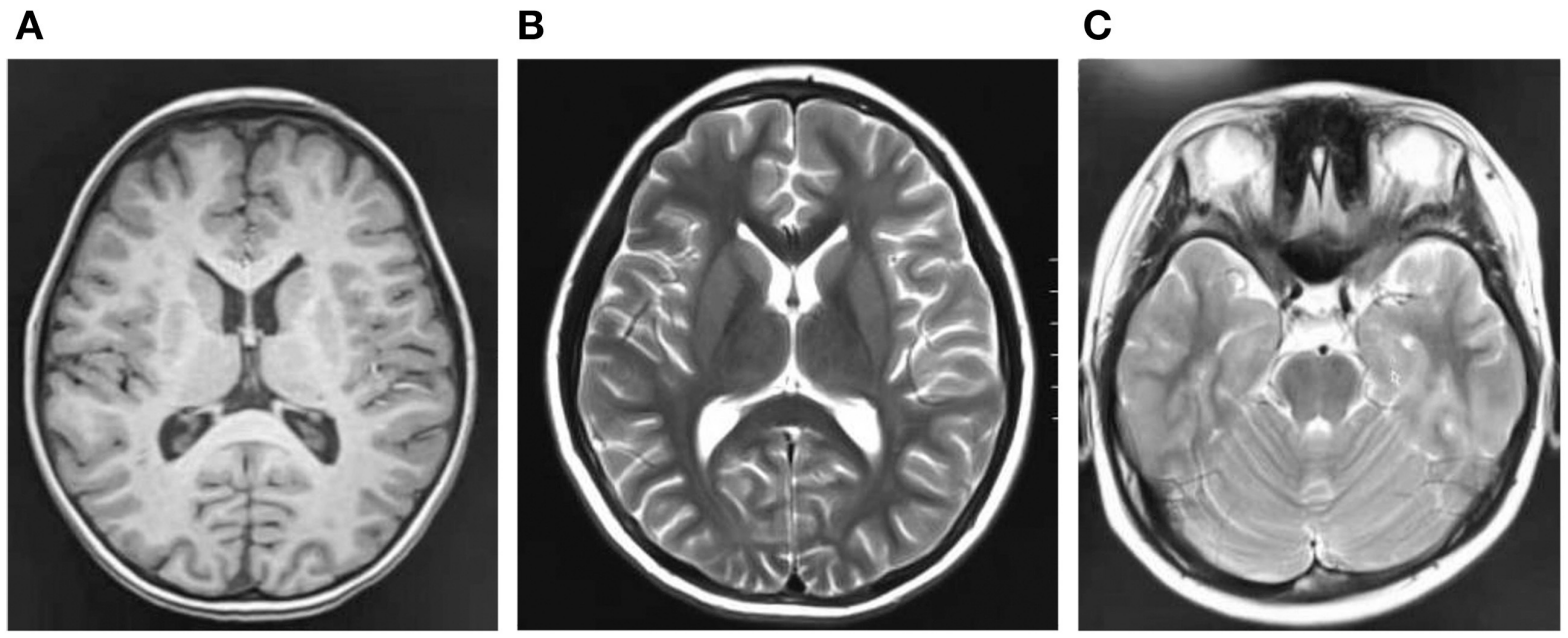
(A–C) Are craniocerebral magnetic resonance imaging of some children, and the results are negative.
